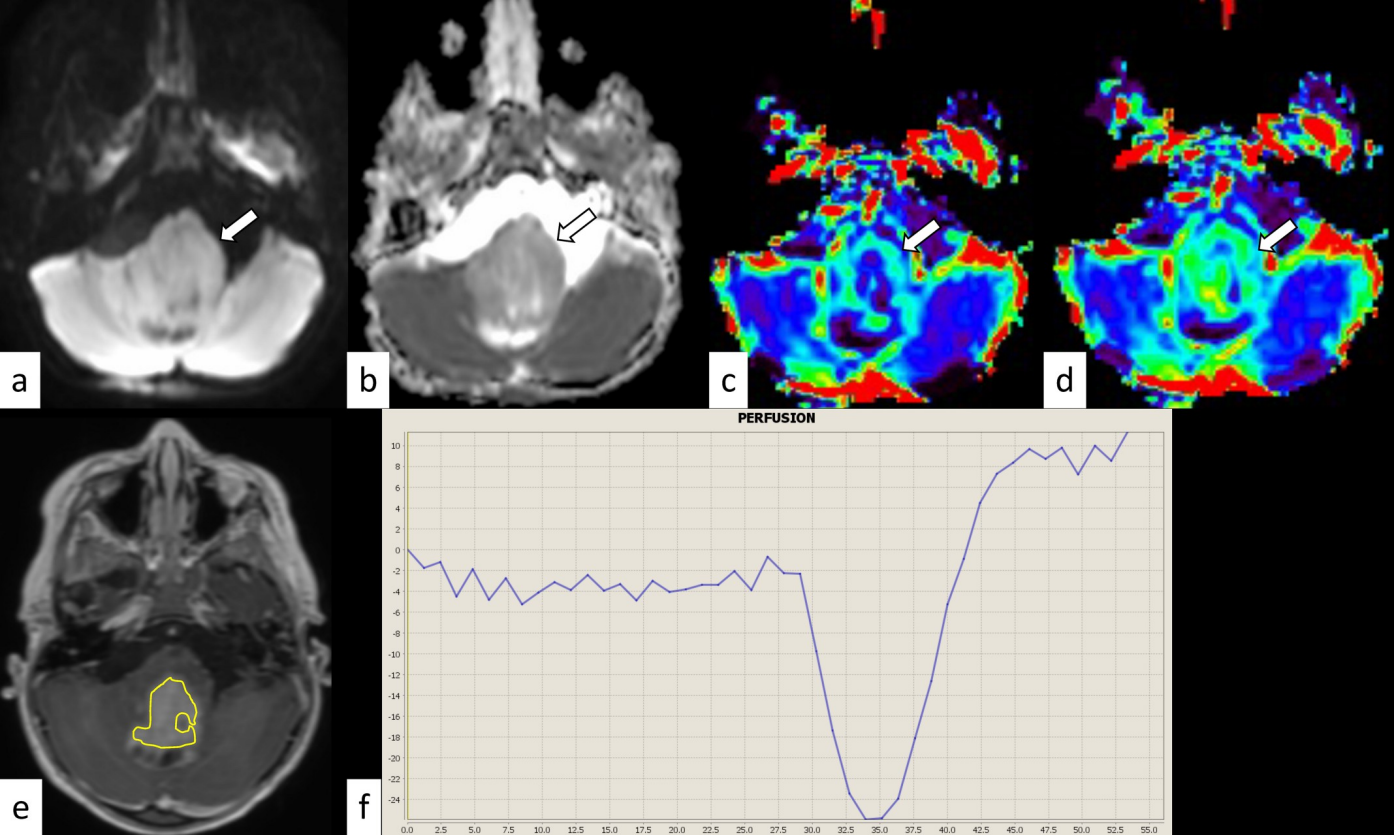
Pontine pilocytic astrocytoma in a 3-year-old boy. The mass shows isointensity on diffusion-weighted imaging (a, arrow) and an increased apparent diffusion coefficient (nADCmean = 1.52) (b, arrow). The normalized relative cerebral blood volume and flow are 1.79 and 1.90, respectively (c and d, arrows). The corresponding post-contrast T1-weighted image and region-of-interest in this slice are shown (e). The percentage signal recovery of the mass is 1.50 (f). nADCmean = normalized mean apparent diffusion coefficient.
